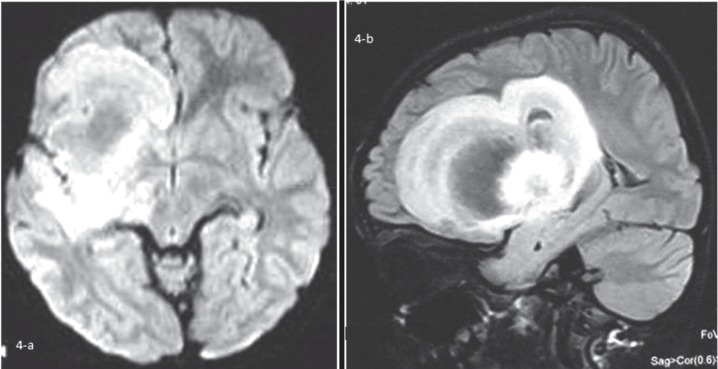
The second brain MRI revealed very large mass like concentric or layered high signal lesion in FLARE sequence in the right frontal lobe white matter and adjacent basal ganglia
